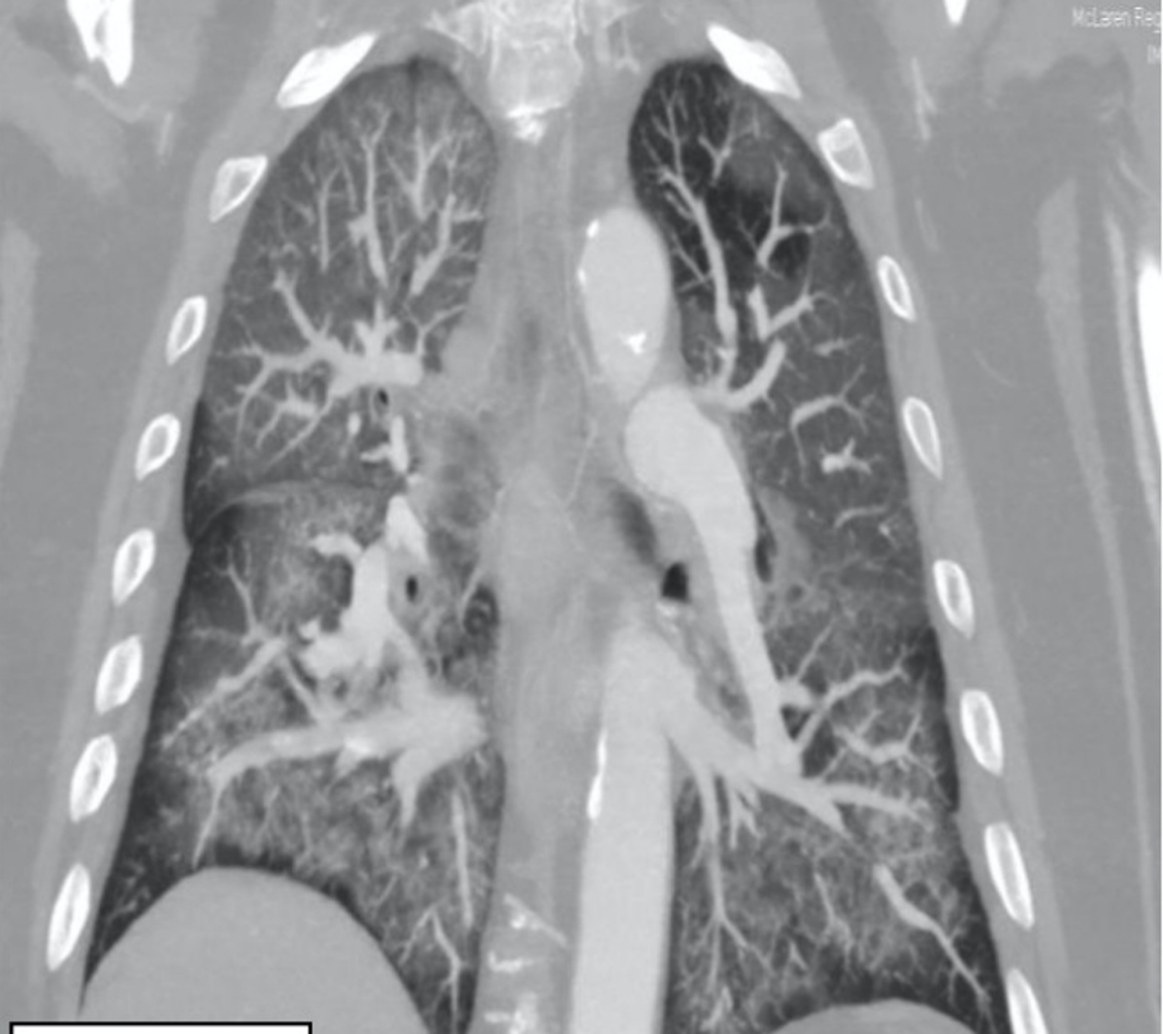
CT chest (coronal view) on second emergency visit showing worsening ground-glass opacities, paratracheal, aorta-pulmonic, and subcarinal lymphadenopathy CT: computed tomography
